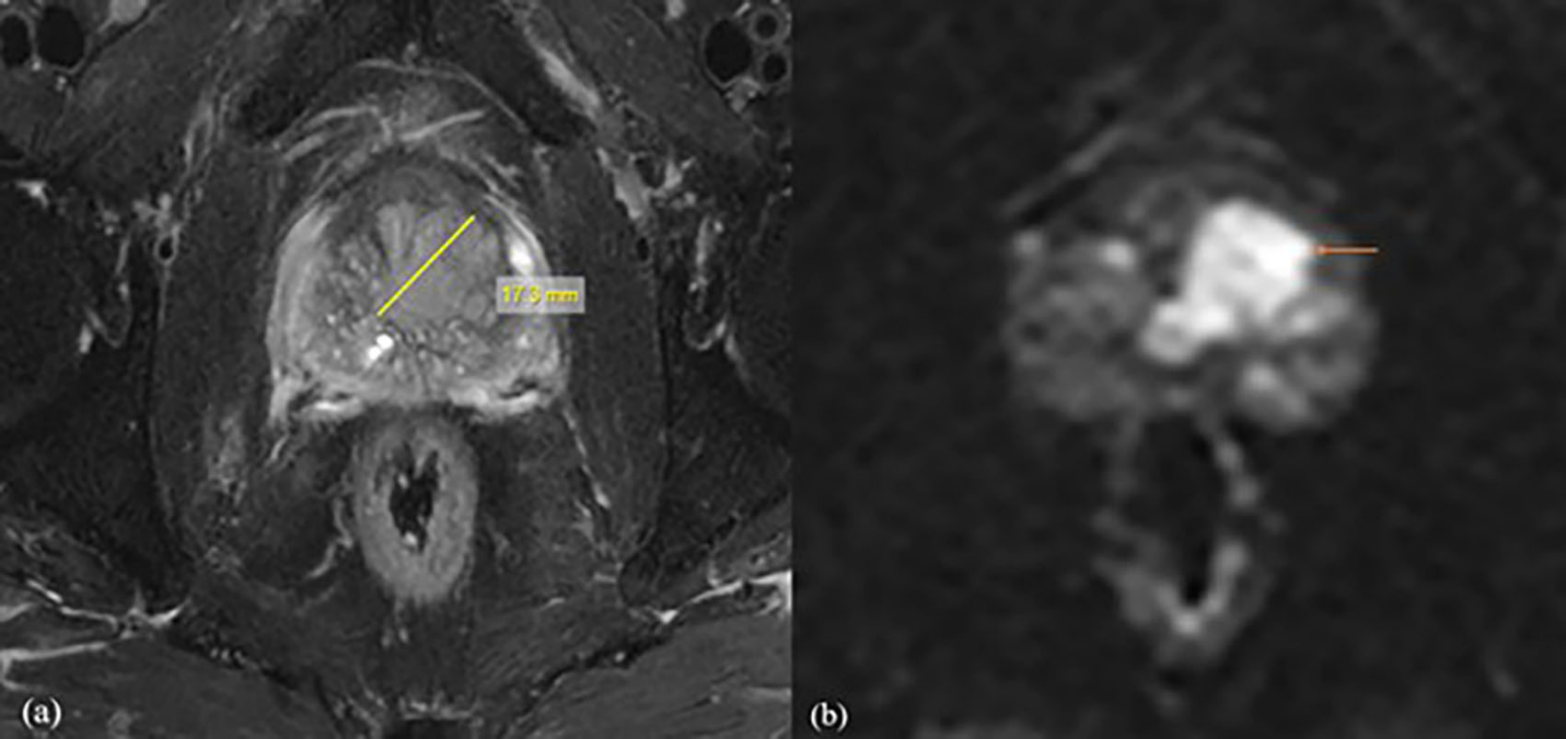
A 72-year-old male patient with difficulty urinating, the biochemistry revealed a PSA level of 58 mg/dl. Following a biopsy, the patient was diagnosed with prostatic acinar adenocarcinoma with a Gleason score of (4+3) = 7. For further investigation, the patient was referred for MRI Prostate. (a) Axial T2 fat suppression sequence showed a lesion with a length of 20.1mm, clearly delineating its boundaries. (b) The DWI with b value of 1500 mm
